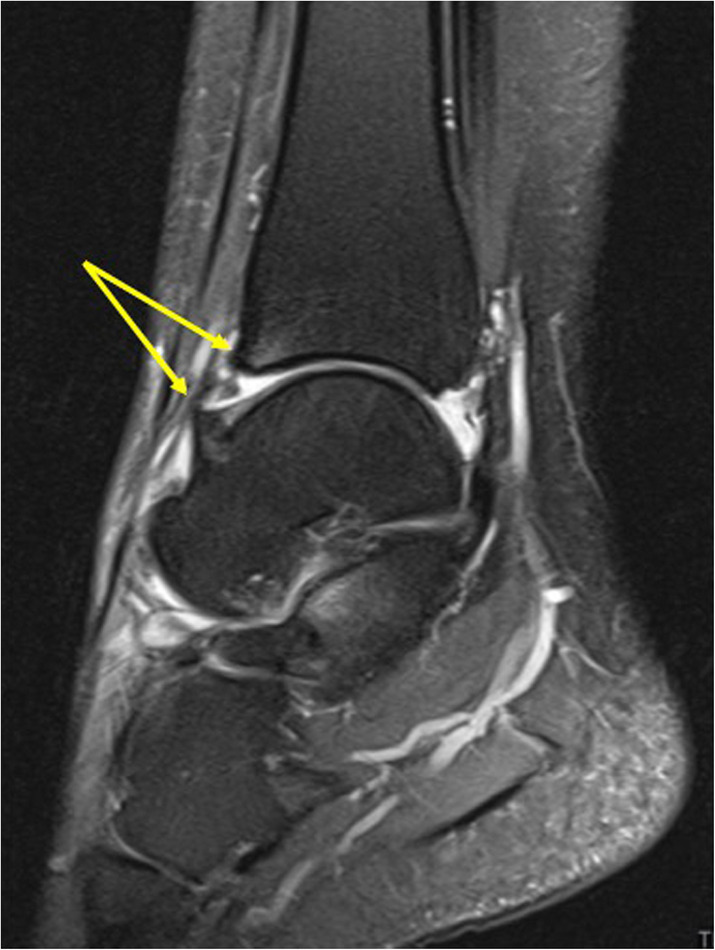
A 22-year-old soccer player presented with chronic anterior ankle pain. There was no history of specific ankle trauma leading to suspicion of anterior impingement. MRI showed anterior impingement with osteophytes (arrows) and bone marrow edema
